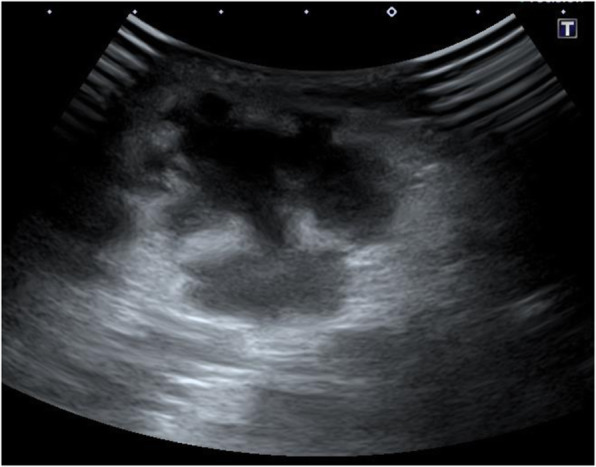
Ultrasonographic imaging: Ultrasonographic image of the affected skin shows a hypoechoic lesion
